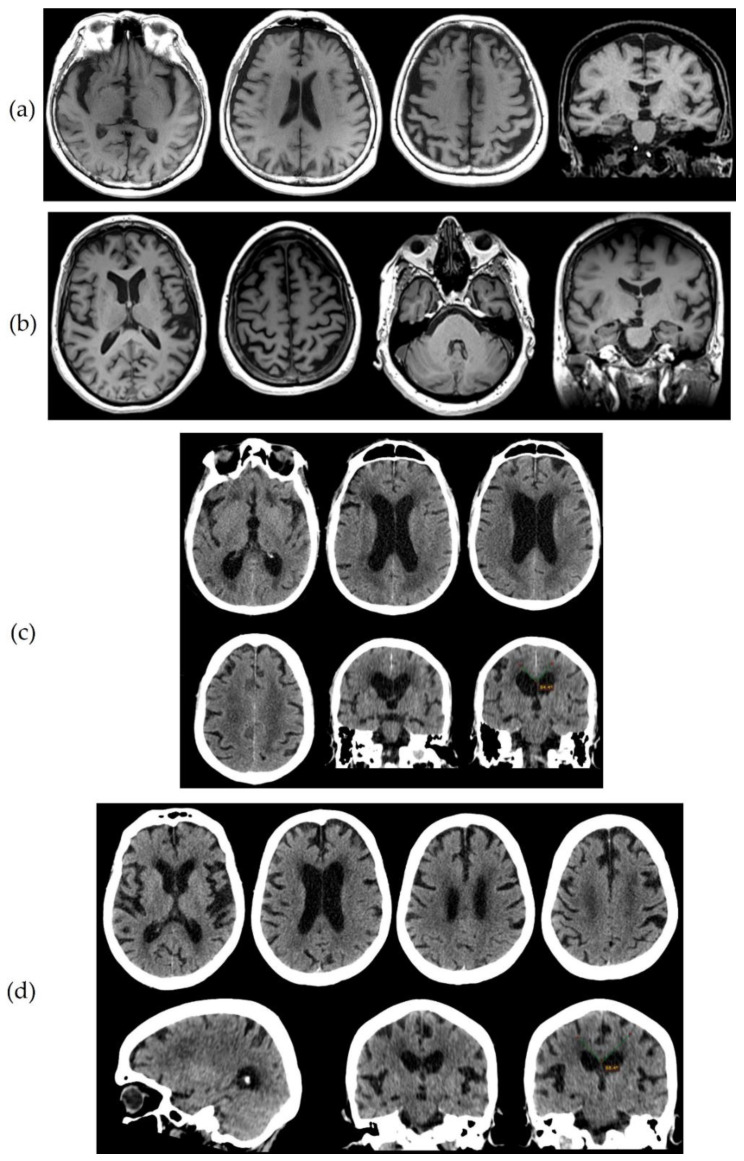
(a) T1 Magnetic Resonance Imaging (MRI) sequences of patient 1. Frontal (mainly), frontoparietal, perisylvian, and left hippocampal (grade 3) atrophy is observed. (b) T1 MRI sequences of patient 2. Atrophy in the left posterior perisylvian and parietal area is observed with preservation of the hippocampus. (c) Computerized tomography (CT) scan of patient 3. Some degree of frontal and parietal atrophy is seen. The white matter shows decreased density consistent with subcortical small vessel disease, in addition to periventricular caps. The parietal convexity is tight, the callosal angle is 84.4° and the Evans index has been calculated to 0.36. (d) CT scan of patient 4. Frontal (mainly) and parietal asymmetric atrophy are observed. Although the parietal convexity is not tight, the callosal angle is 88.4° and the Evans index has been calculated to 0.38. Decreased density of the white matter at centrum semiovale is noted, consistent with small vessel disease, with additional periventricular caps.
